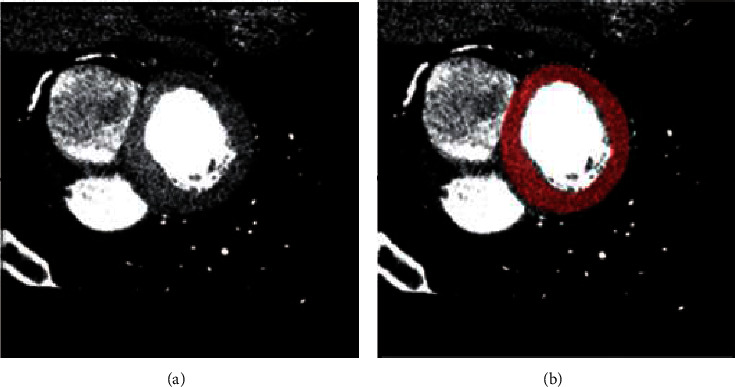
CT scan of LV (a) and LV boundary (b).
